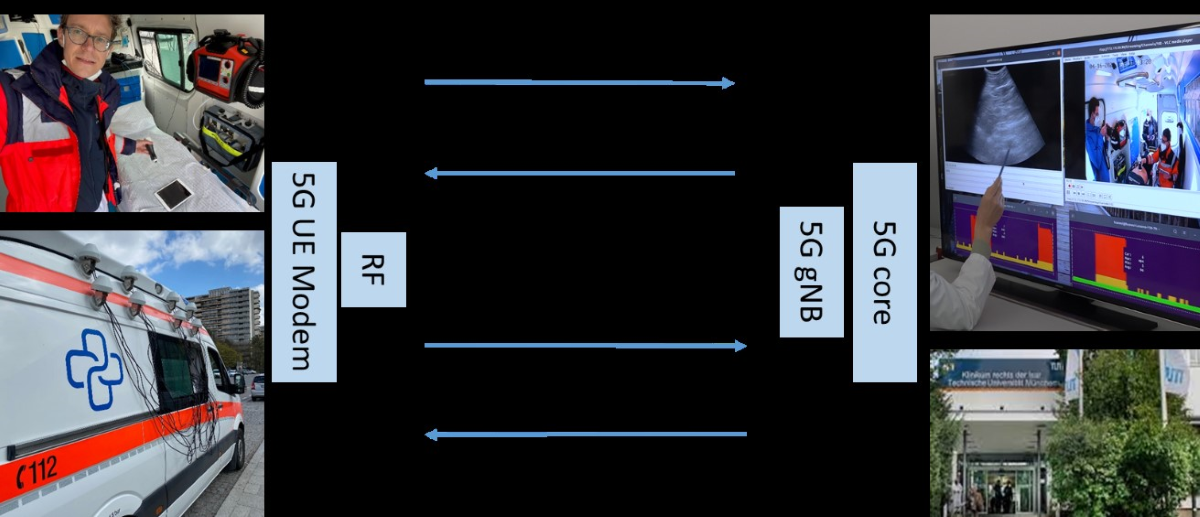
Framework for the 5G field test. Ambulance car (left) equipped with mobile ultrasound and pan, tilt, zoom camera connected to a UE modem. The latter is connected to an RF unit, which is connected to UE antennas on the top roof of the ambulance car. The remote hospital site (right) is connected to the 5G core, which is connected to the gNB. gNB: gNodeB; RF: radio frequency; UE: user equipment.
